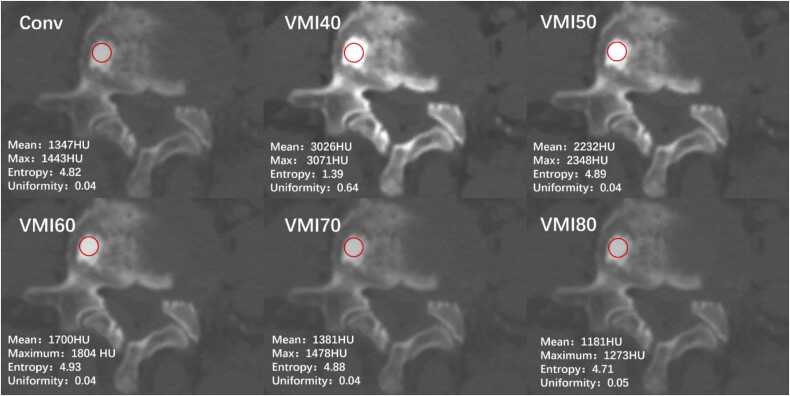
Axial CT images in an 87-year-old female patient with BI lesion. Maximum, minimum, entropy, and uniformity of lesions measured on conventional images and VMI40–80. BI, Bone island; CT, computed tomography.
